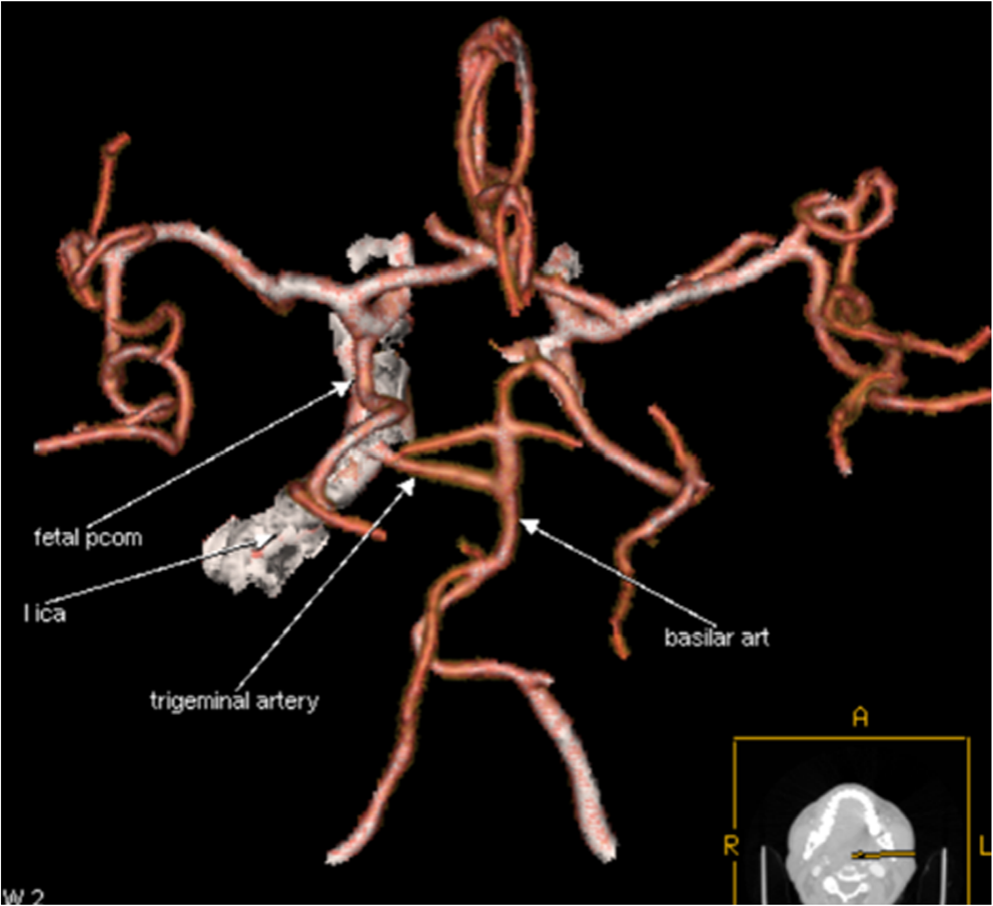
CTA (VRT 3D reconstructions) shows a left-sided trigeminal artery joining the basilar artery. The basilar artery (which is relatively hypoplastic, proximal to the connection site) gives rise to the right PCA, while the left PCA arises from the ICA (foetal PCom) (Saltzman’s type II or Weon’s type 3) (annotations)
